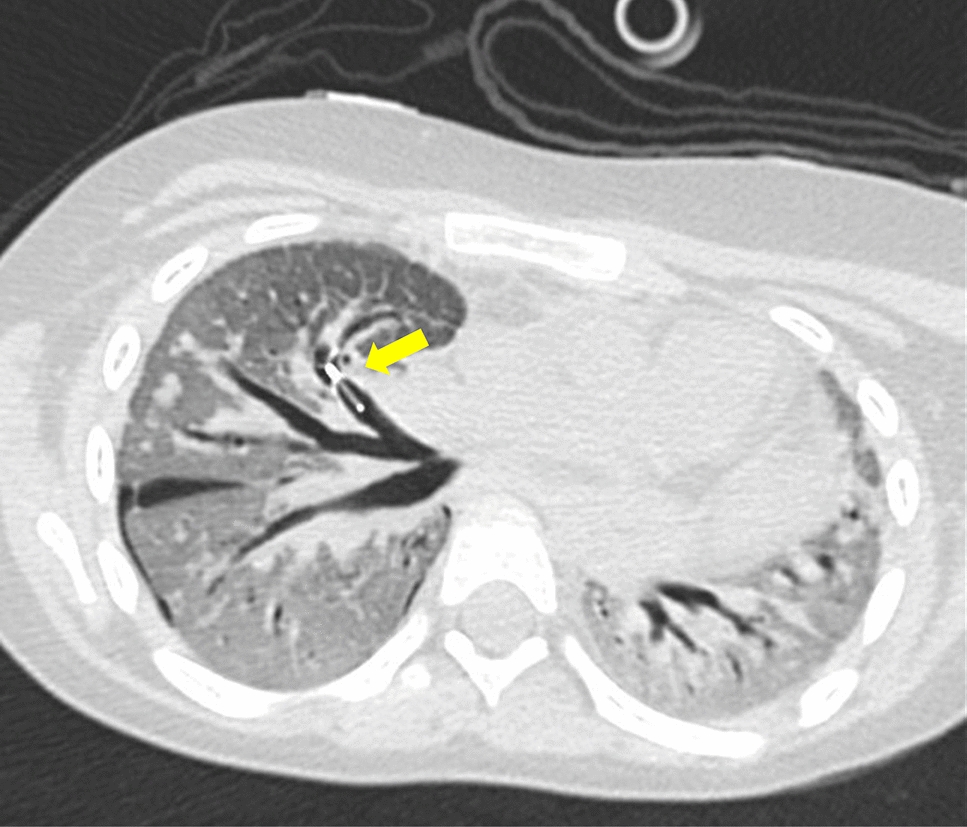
Computed tomography chest image with endobronchial valve in view (yellow arrow)
